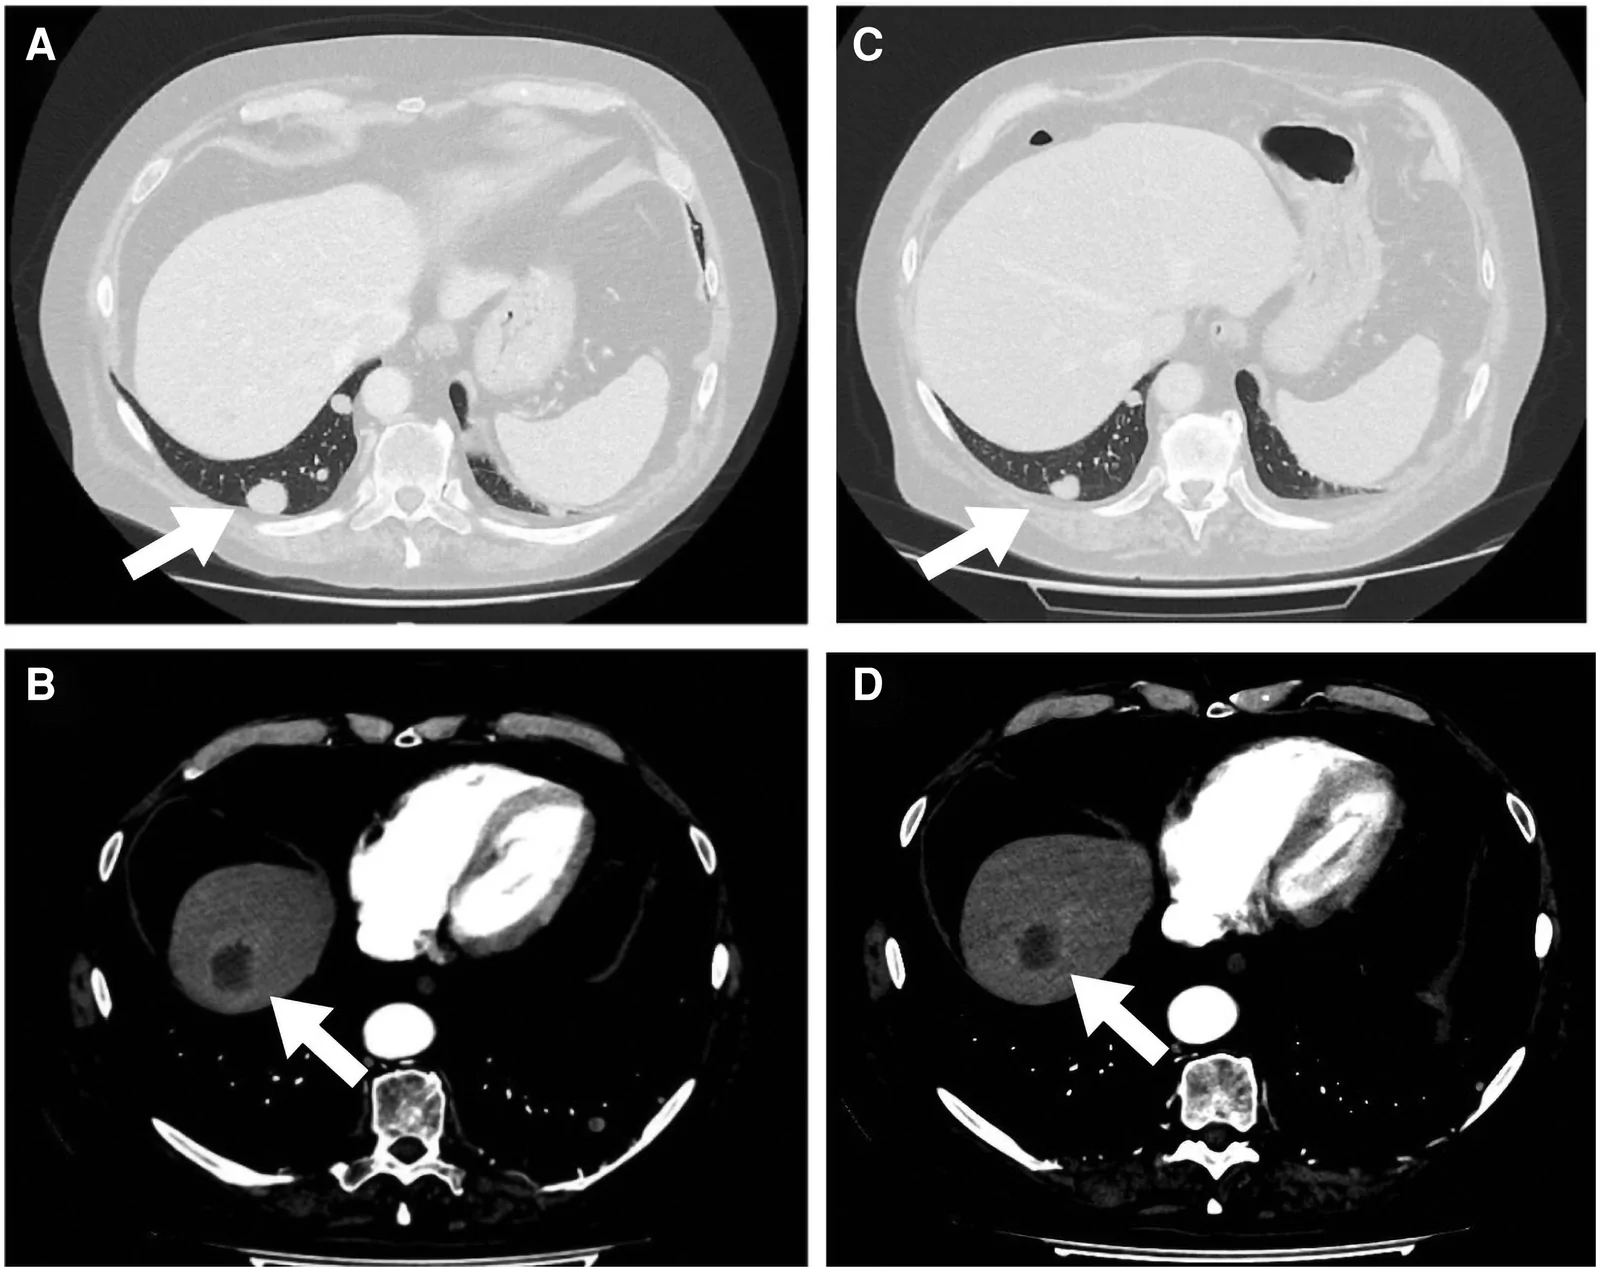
Comparison of lung and liver metastasis between the computed tomography scan of October 2024 (A and B) and the computed tomography scan of February 2025 (C and D).
